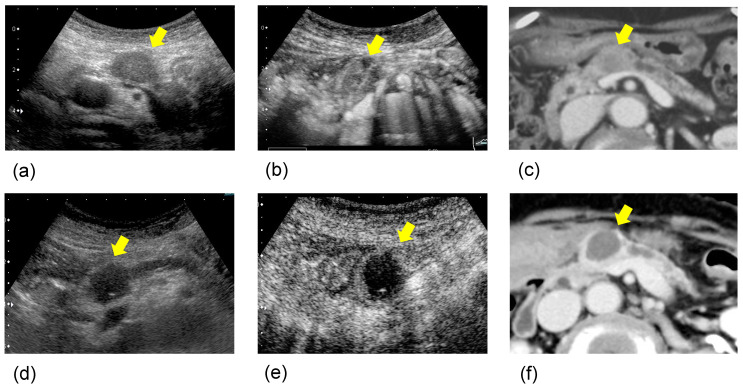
A case with a better prognosis after HIFU therapy: The patient was an 83-year-old woman with pancreatic body cancer 20 mm in size. (a) B-mode ultrasonography (US) (pre HIFU); the pancreatic body tumor showed hypoechogenicity in B-mode US. (b) Contrast-enhanced ultrasonography (CEUS) (pre HIFU); the tumor showed isovascular on CEUS. (c) Contrast-enhanced computed tomography (CECT) (pre HIFU); the tumor showed low-density area in CECT. (d) B-mode US (post HIFU); after one session of HIFU therapy, the tumor showed echogenic changes in B-mode US. (e) CEUS (post HIFU); the tumor showed hypovascular changes on CEUS. (f) CECT (post HIFU); it showed a low-density area with no contrast effect, and complete coagulative necrosis was observed. Thereafter, she survived for 2 years without active treatment.
